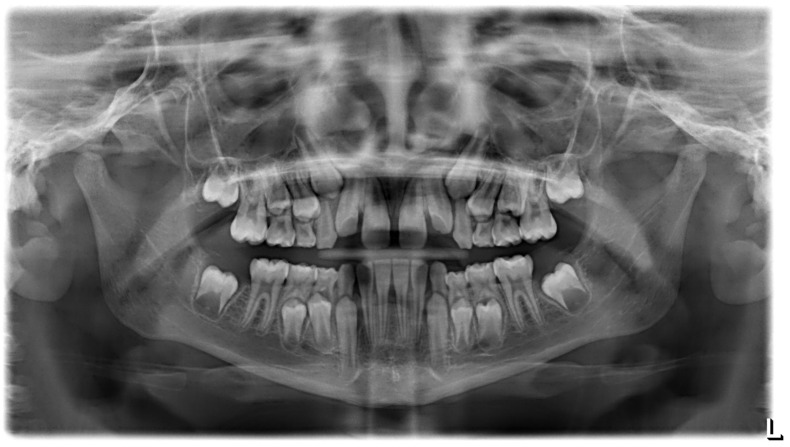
Radiographs of the subject at the end of the treatment. Orthopantomography X-ray after treatment (8-year-old).
